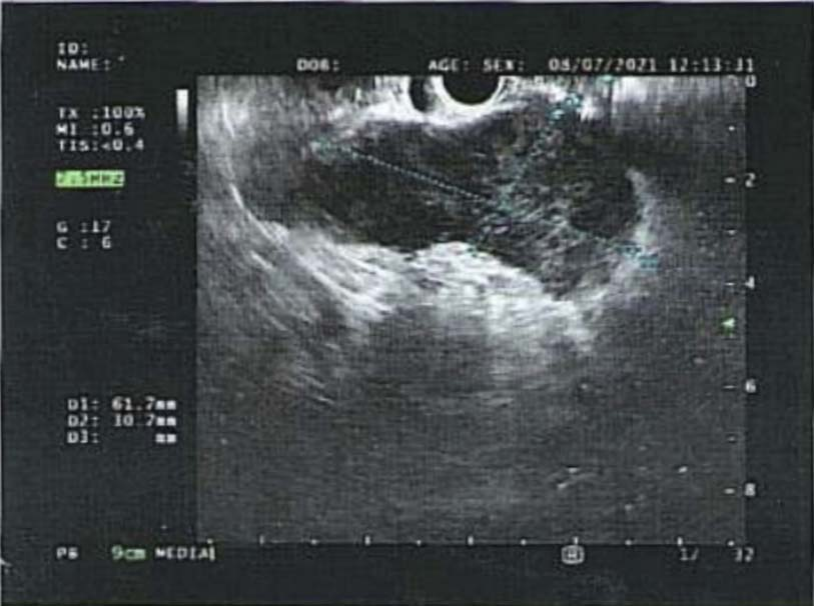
Endoscopic Ultrasound of the abdomen showing bulky adrenal gland.
